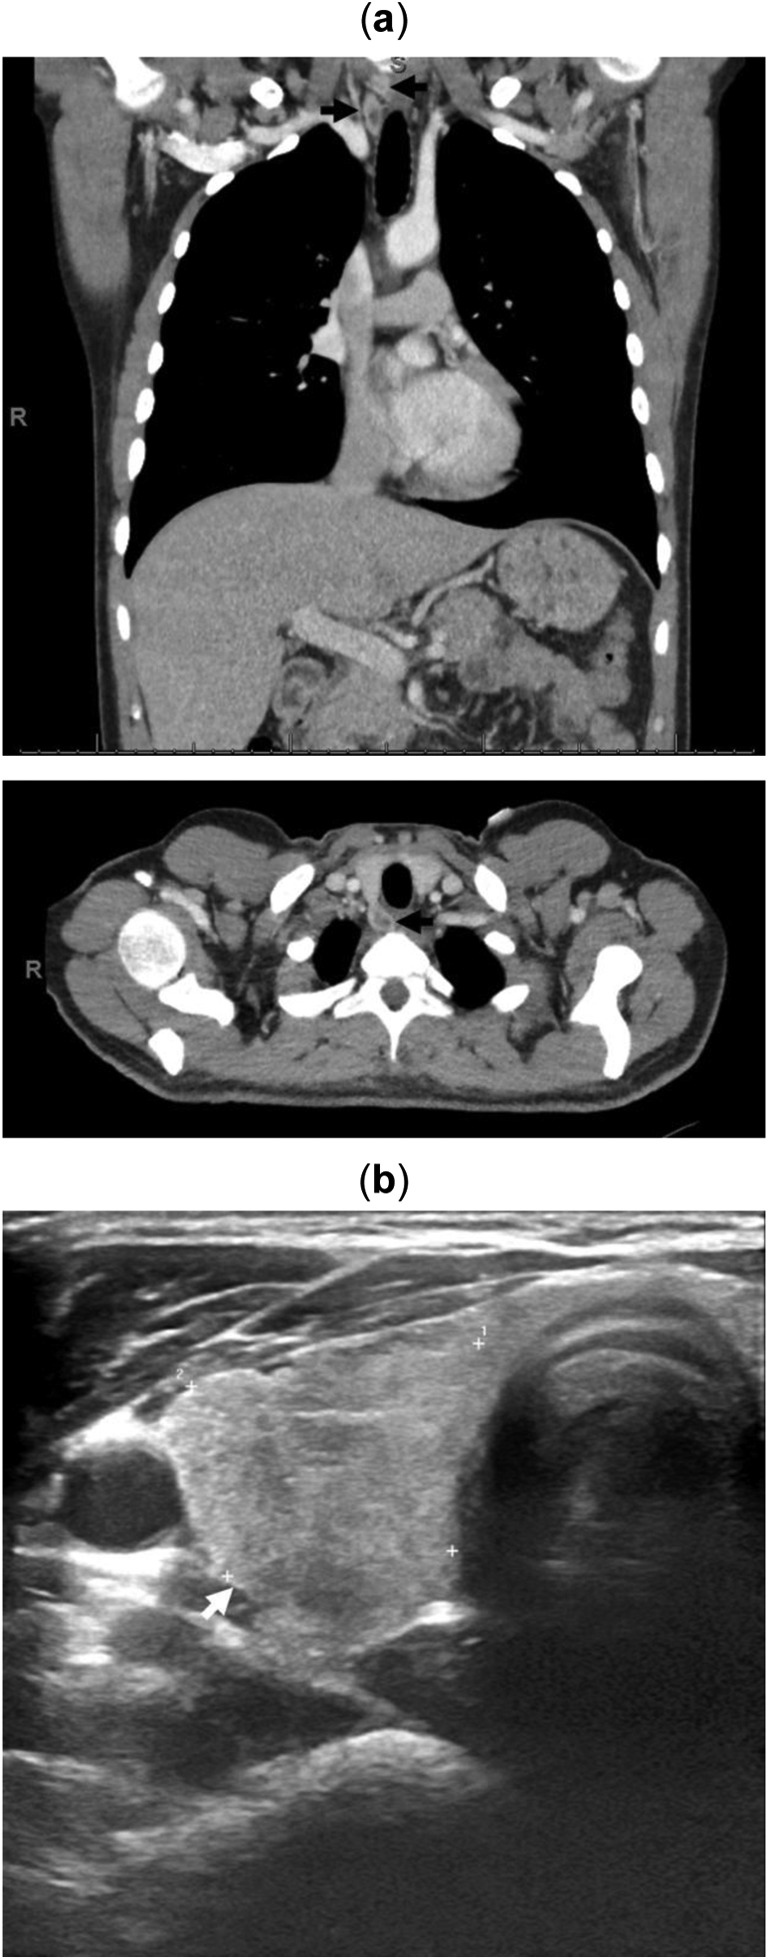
Two parathyroid adenomas identified by CT (a) and ultrasound scan (b) located posterior and inferior to the right thyroid lobe in a young man (case 5)
